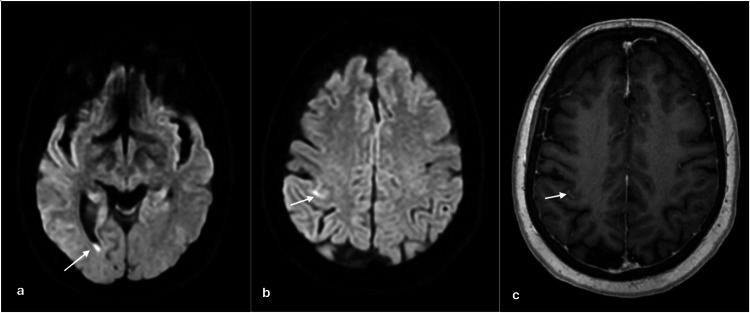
MRI of the brain with and without IV contrast revealing an abnormal diffusion restriction signal within the occipital horn of the right lateral ventricle (a), diffusion restriction signal abnormality within the sulci of the right cerebral hemisphere (b) with corresponding FLAIR hyperintensity, and abnormal enhancement (c) suggestive of purulent material MRI: magnetic resonance imaging; IV: intravenous; FLAIR: fluid-attenuated inversion recovery
